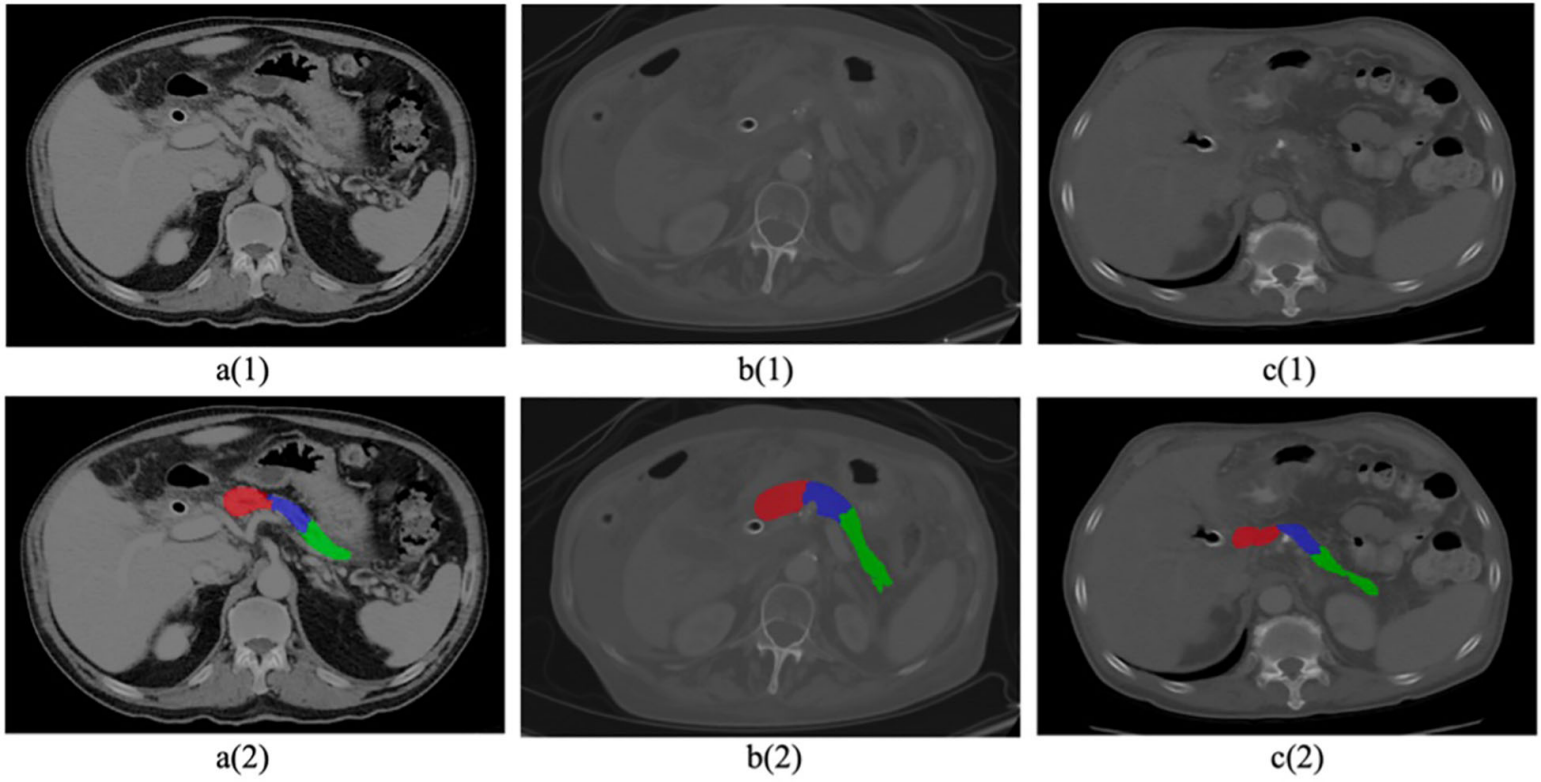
Sample CT images with cancerous pancreas. Top row 1 (a–c) shows original raw images, Bottom row 2 (a–c) shows segmented pancreas and subregions (head in red, body in blue, tail in green).
